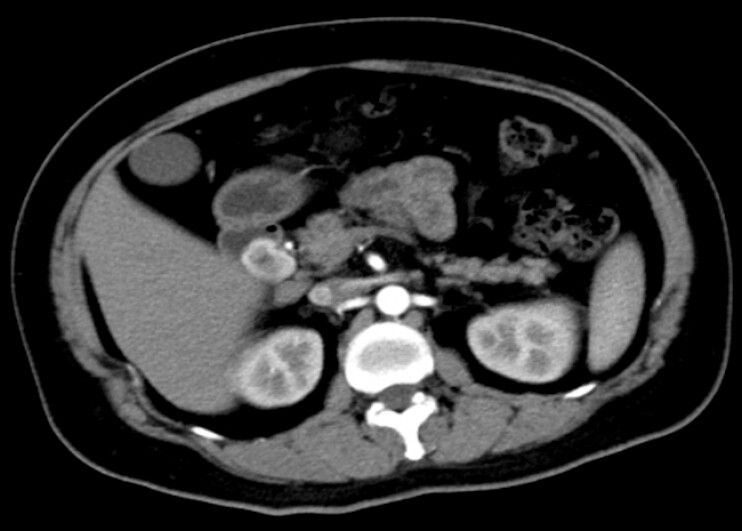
Enhanced computed tomography showed a round-like abnormal enhancement in the duodenal bulb.
